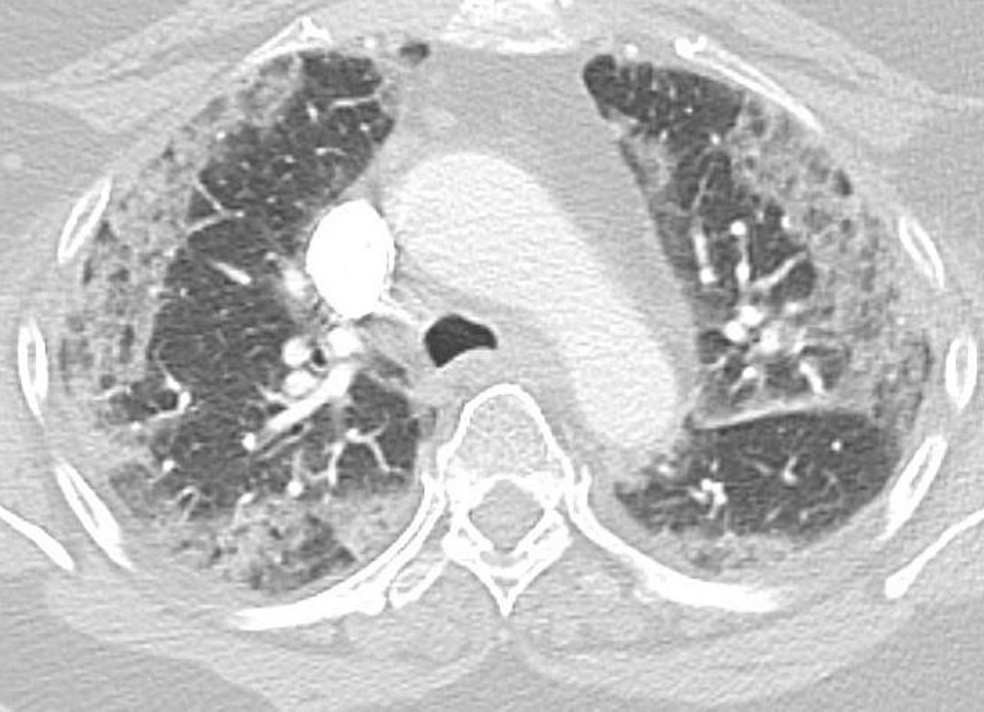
Admission chest CT scan showing bilateral peripheral ground-glass opacities
